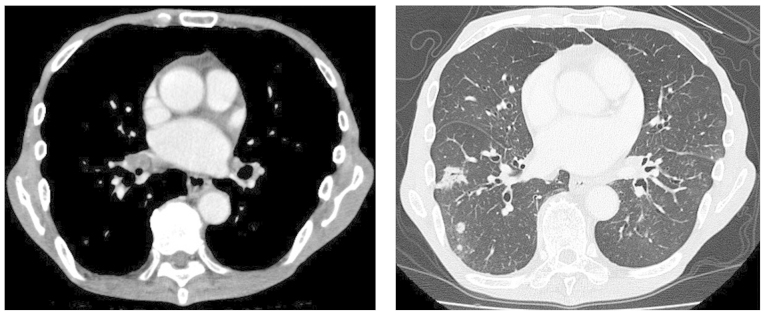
A chest computed tomography scan revealing an ill-defined mass in each lung, with bilateral hilar lymph node swelling.
